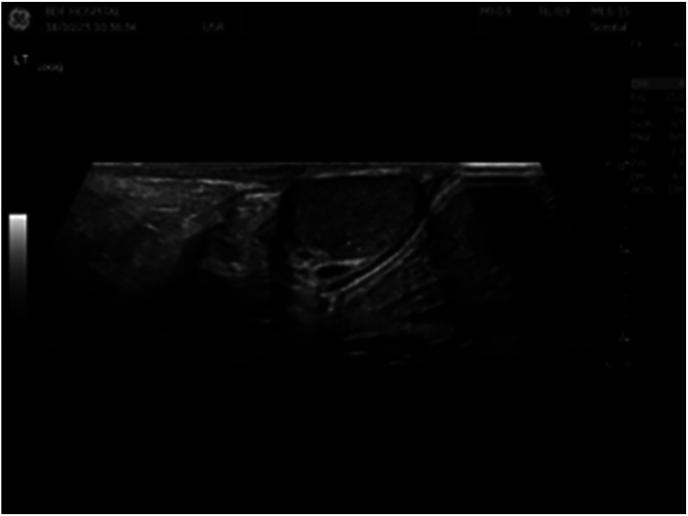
Microlithiasis on ultrasonography of testes.
